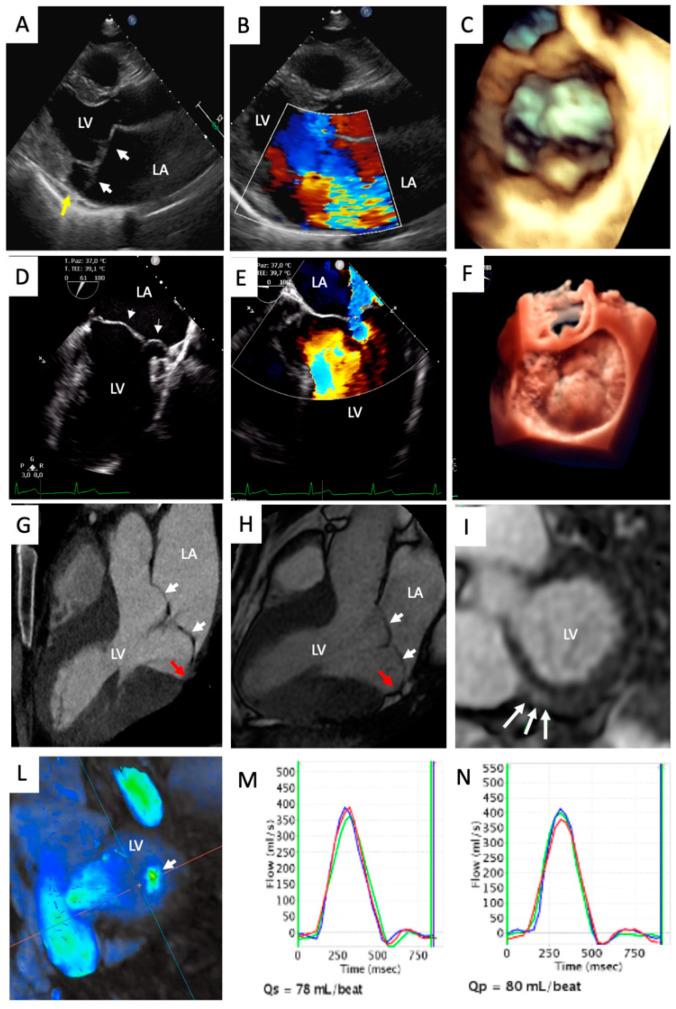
Multimodality MVP assessment in a 37-year-old male symptomatic for exercise dyspnea. (A) TTE PLAX view showing a myxomatous MV with bileaflet MVP (white arrows) with associated MAD (yellow arrow); (B) ColorDoppler TTE PLAX view showing centrovalvular mitral regurgitation jet; (C) 3D-TTE demonstrating bileaflet MVP; (D) bicommisural TEE images confirming bileaflet MVP (white arrows); (E) TEE 4-Ch view, assessment of mitral regurgitation severity measuring the PISA radius; (F) 3D-TEE with photo-realistic 3D rendering confirming bileaflet MVP; (G,H) CCT (G) and CMR (H) confirming the presence of bileaflet MVP (white arrows) with associated MAD (red arrow); (I) CMR late gadolinium enhancement images showing focal fibrosis of the LV inferobasal wall; (L) four-dimensional (4D)-flow CMR basal short axis view showing centrovalvular mitral regurgitant jet; (M,N) phase contrast measurements of aortic (M) and pulmonary (N) flow. CCT: Cardiac CT; CMR: Cardiac Magnetic Resonance; MV: Mitral Valve; MAD: Mitral Annular Disjunction; MVP: Mitral Valve Prolapse; PISA: Proximal Isovelocity Surface Area; PLAX: Parasternal Long Axis; TEE: Transesophageal echocardiography; TTE: Transthoracic Echocardiography.
